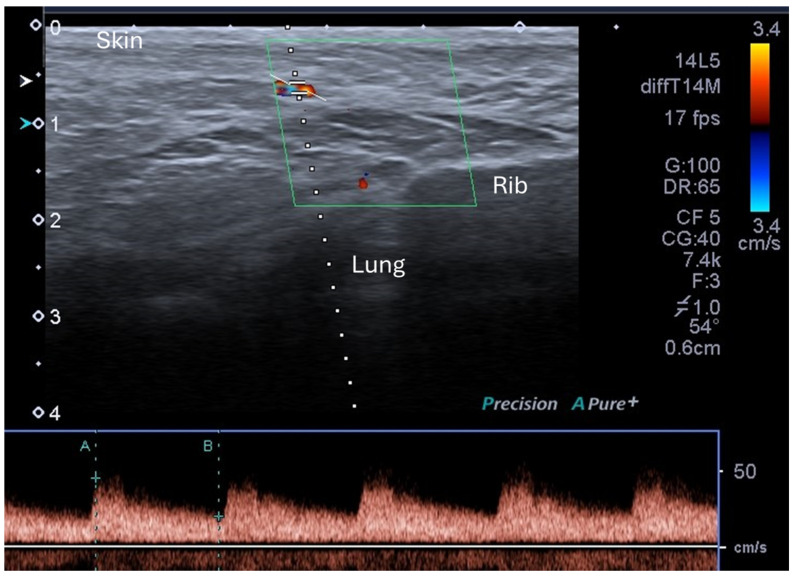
Colour flow Doppler imaging of the internal mammary artery visualised as the coloured vessel within the green box. The colours represent velocity of the blood flow. A, peak systolic velocity; B, end diastolic velocity as measured by pulsed wave doppler sampled within the internal mammary artery.
